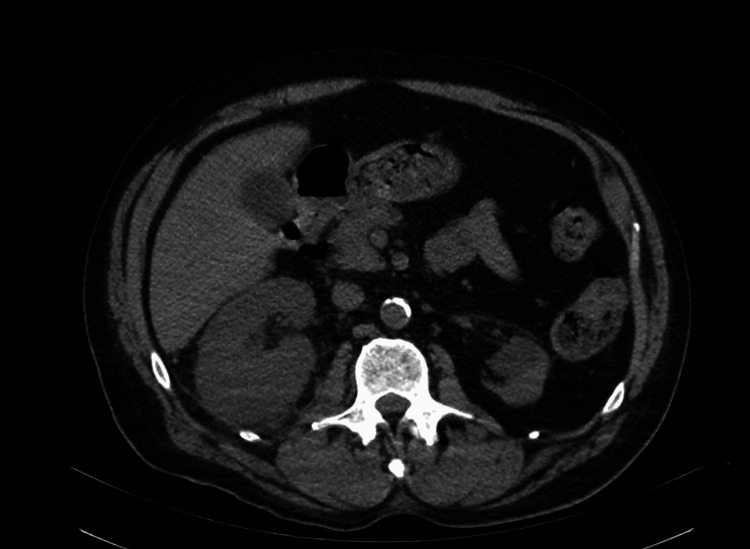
CT of the abdomen/pelvis with contrast showed evidence of acute right pyelonephritis
